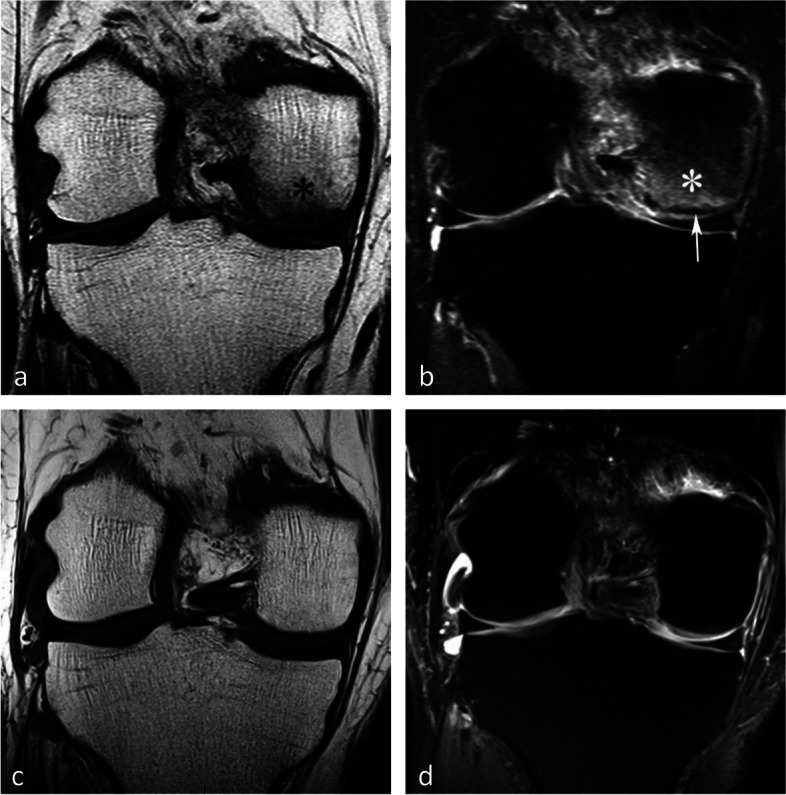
Borderline thickness of bone marrow low signal on T2-weighted image. a, b MRI shows low signal intensity on T1-weighted (a) and high signal intensity on fat-suppressed T2-weighted images in the medial femoral condyle (b) (asterisks). Immediately near the subchondral surface, fat-suppressed T2-weighted image shows a very thin layer of tissue of borderline thickness with low signal intensity (arrow in b). c, d In the present case, the follow-up at 3 months showed healing, with normalization of the signal in the inferior pole of the condyle
